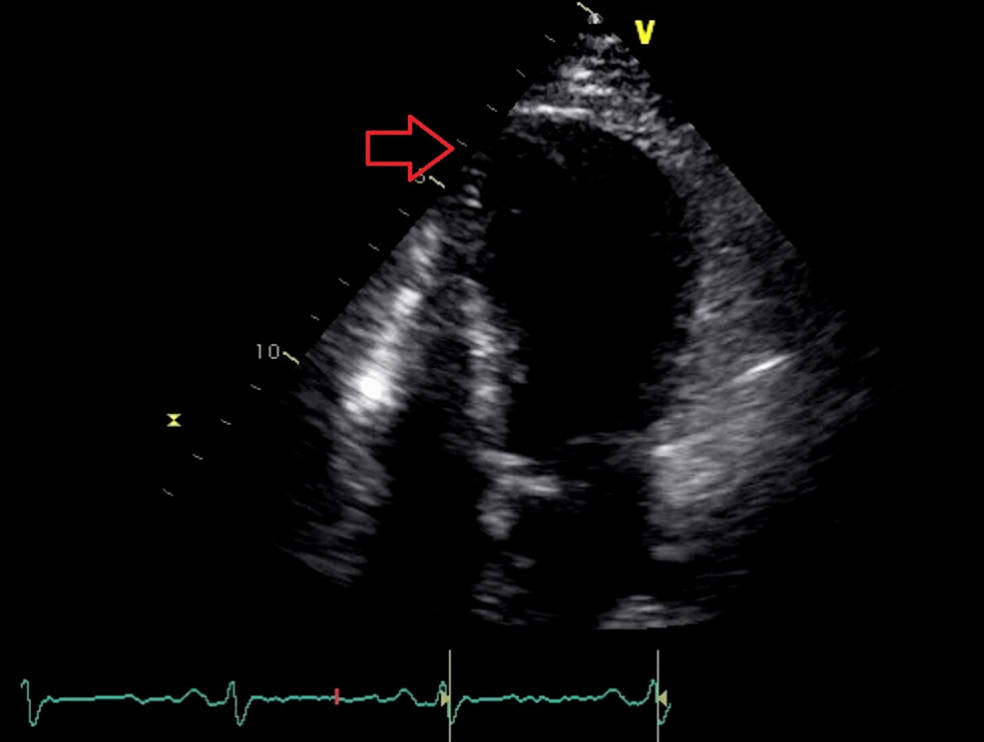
Transthoracic echocardiogram showed apical hypokinesia with ballooning (red arrow)
